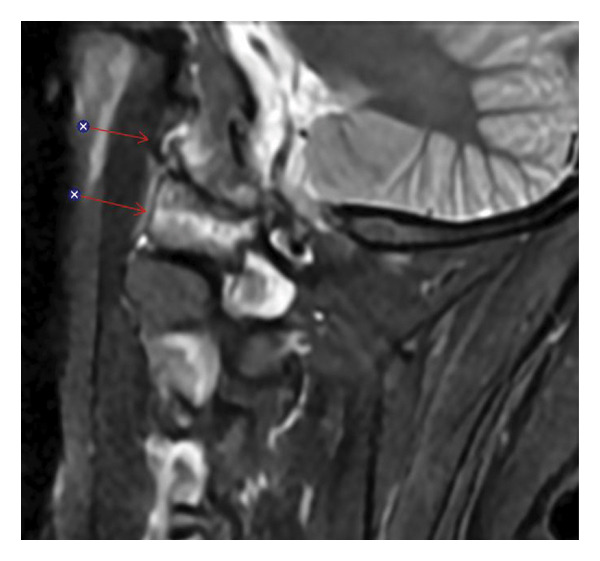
Sagittal STIR MRI of the upper cervical spine showing bone marrow edema around the left atlanto‐occipital joint with associated hyperintense signal in the adjacent soft tissues (arrows).
